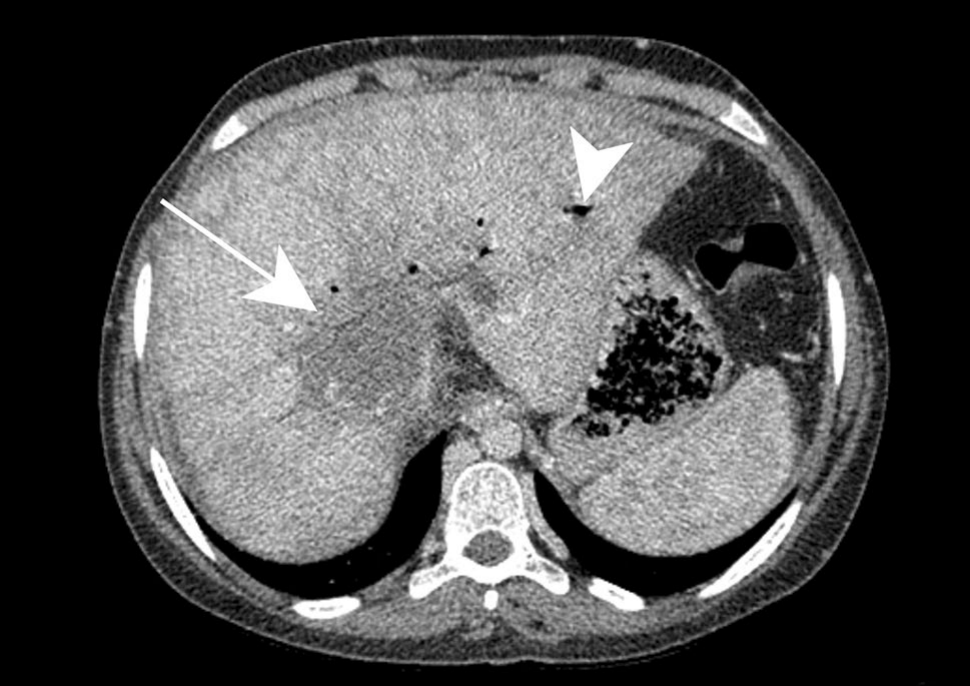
Budd-Chiari syndrome secondary to compression of liver out-flow by the hydatid cyst in a 20-year-old female patient. Hypertrophy of the caudate lobe with inhomogeneous mottled liver appearance is indicative of Budd-Chiari syndrome. Axial delayed phase contrast-enhanced CT shows a deformed cystic lesion in the deep portion of segments VII and VIII of the liver (white arrow) associated with pneumobilia secondary to previous sphincterotomy (white arrowhead)
